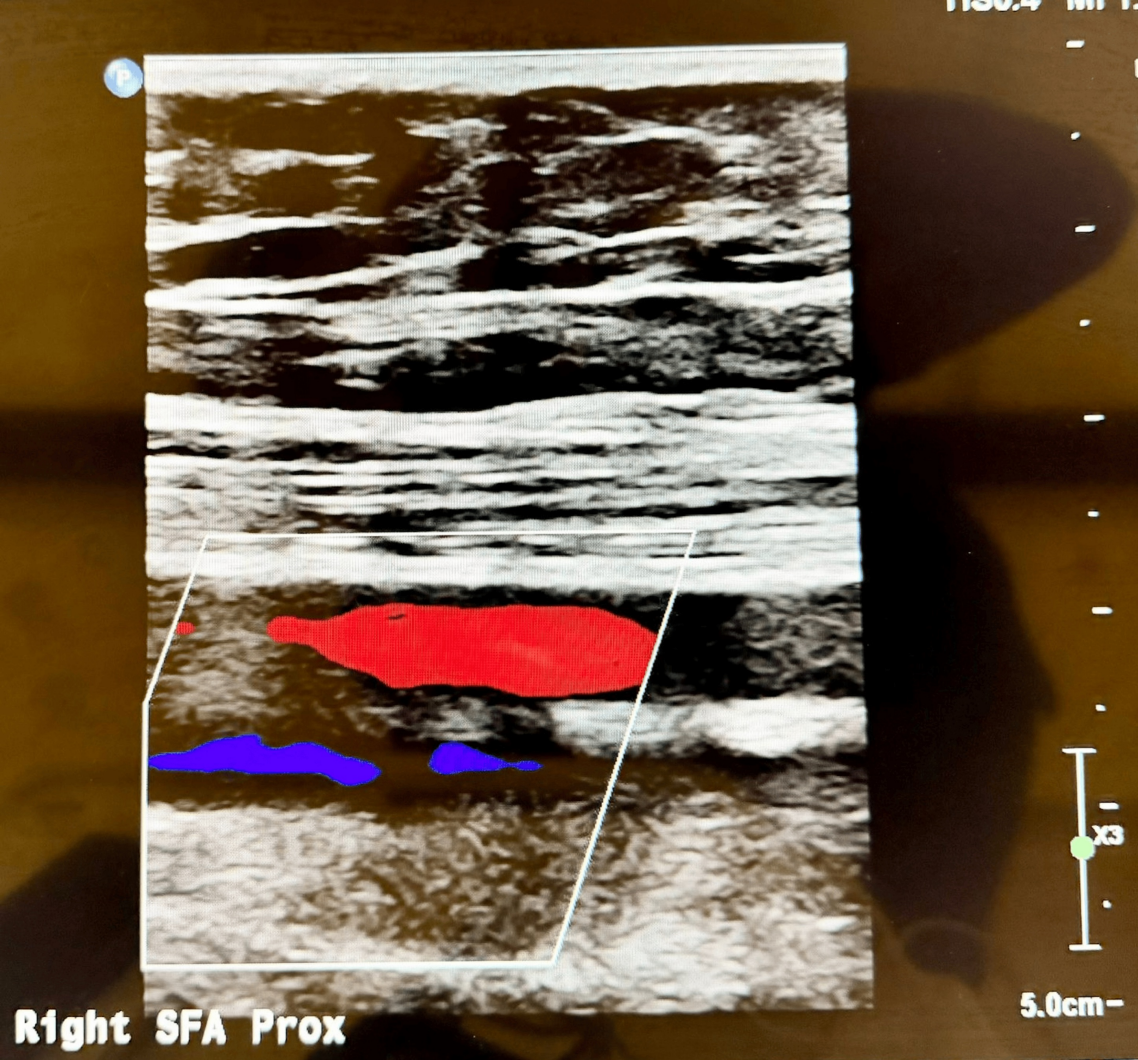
Normal Peripheral Arterial Ultrasound Grayscale and colour Doppler ultrasound demonstrating normal peripheral anatomy with preserved vessel caliber, intact wall morphology, and normal laminar blood flow. No aneurysmal dilatation, stenosis,or intraluminal thrombus was identified.
